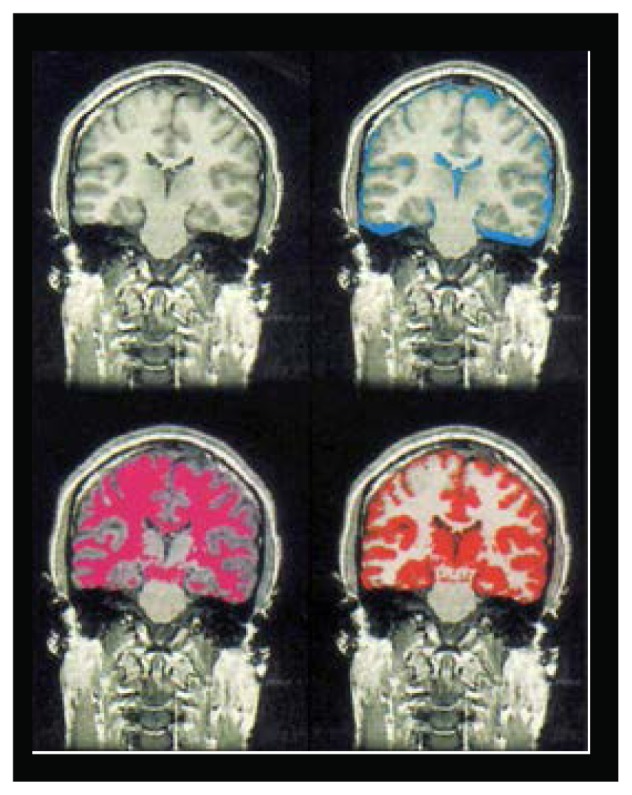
Magnetic resonance imaging can provide more detailed structural information about the brain than is obtainable using computed tomography. Image courtesy of Daniel Hommer.
